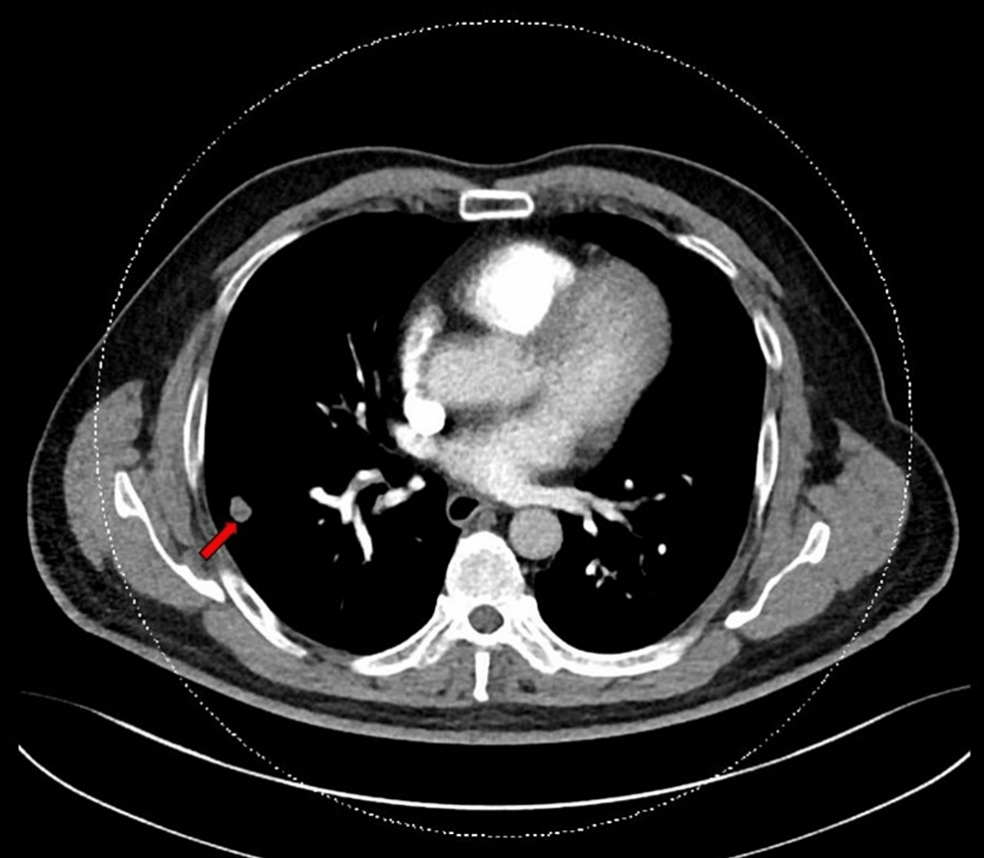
Computed tomography of the thorax showing the mildly lobulated right nodule.
